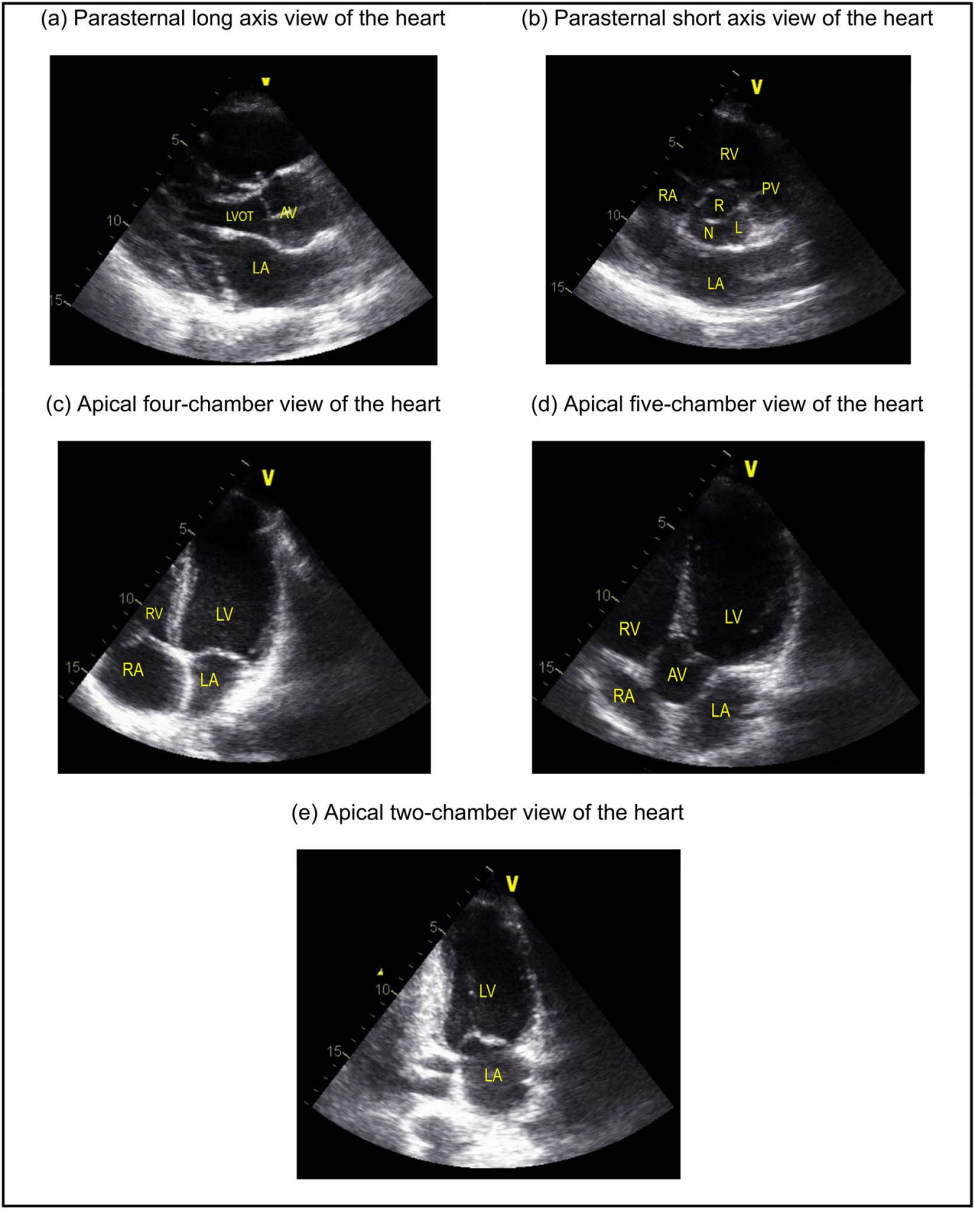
Views of heart used for Doppler echocardiography measurements. (a) Parasternal long axis view of the heart: blood enters the left ventricle through the left atrium, exiting through the left ventricular outflow tract leading to the aortic valve; (b) Parasternal short axis view of the heart: the aortic valve leaflets are shown opening and closing. Above the aortic valve is the right ventricle, through which blood exits the right ventricular outflow tract into the pulmonary artery; (c) Apical four-chamber view of the heart: right atrium opens into the right ventricle, and the left atrium opens into the left ventricle simultaneously; (d) Apical five-chamber view of the heart: mitral valve allows blood to enter the left ventricle, then exit through the aortic valve; (e) Apical two-chamber view of the heart: blood moves from the left atrium, through the mitral valve, into the left ventricle. Abbreviations: LVOT: left ventricular outflow tract; AV: aortic valve; LA: left atrium; RV: right ventricle; RA: right atrium; PV: pulmonary valve.
